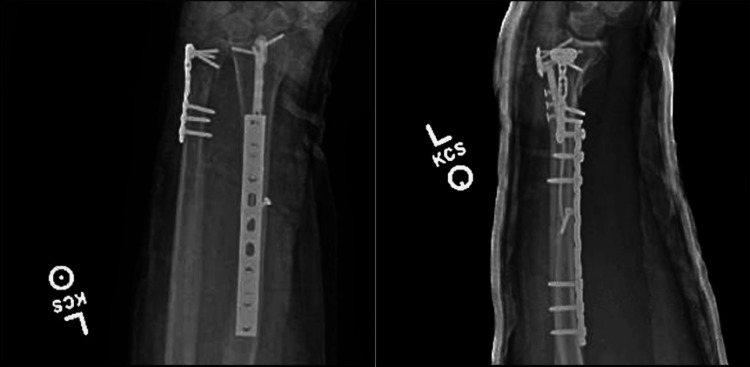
Anteroposterior (A) and lateral (B) X-ray films demonstrating restoration of anatomic alignment of the peri-implant fracture of the left distal radius using a 2.7 mm lag screw and a nine-hole 3.5 mm limited-contact dynamic compression plate The left distal ulna fracture was restored with a contoured 2.7 mm T-plate. A sugar-tong splint is in place.
